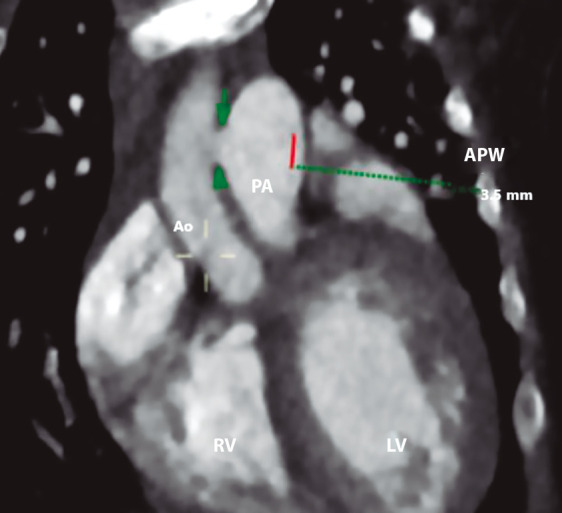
Coronal view of computed tomography showing the location of the aortopulmonary window. Delineation and measurement of the aortopulmonary window (green arrow). APW: Aortopulmonary window. Ao: Aorta. PA: Pulmonary artery. RV: Right ventricle. LV: Left ventricle.
